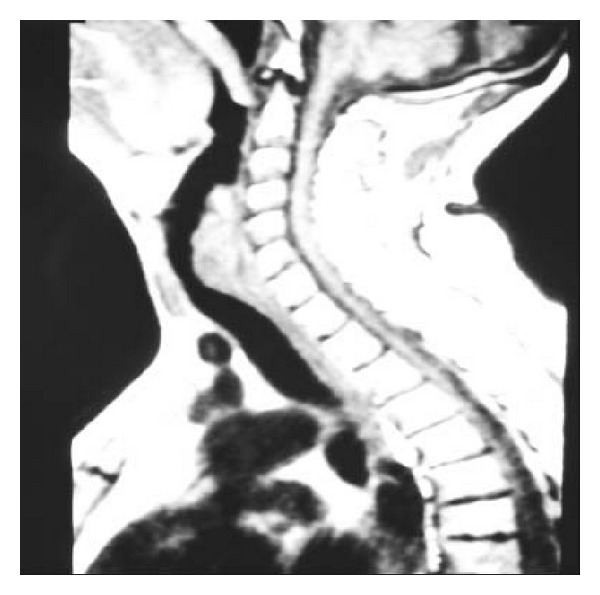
MRI of neck. Laryngeal mass, starting from the region of the left vocal cord and expanding in the trachea until the level of 4th thoracic vertebra.
